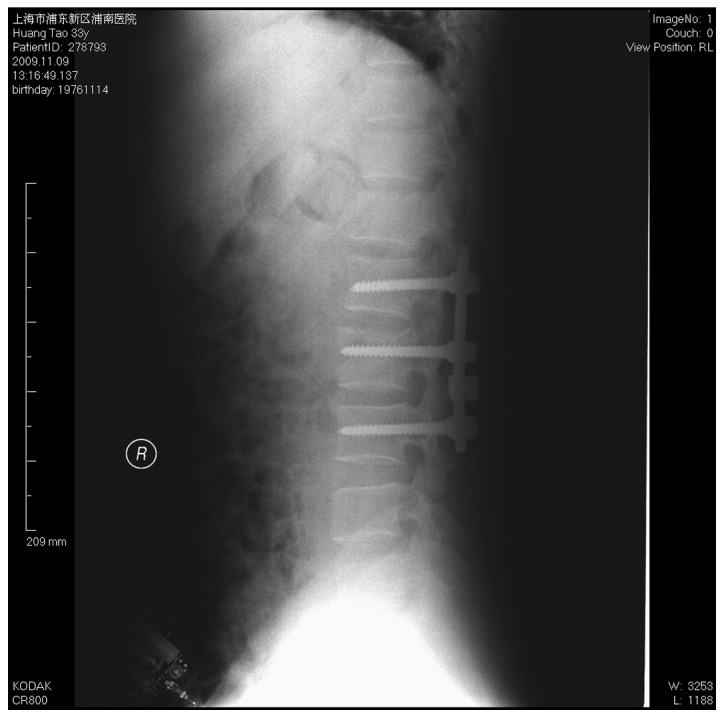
Lateral radiograph following triplane fixation of an L2 fracture.
